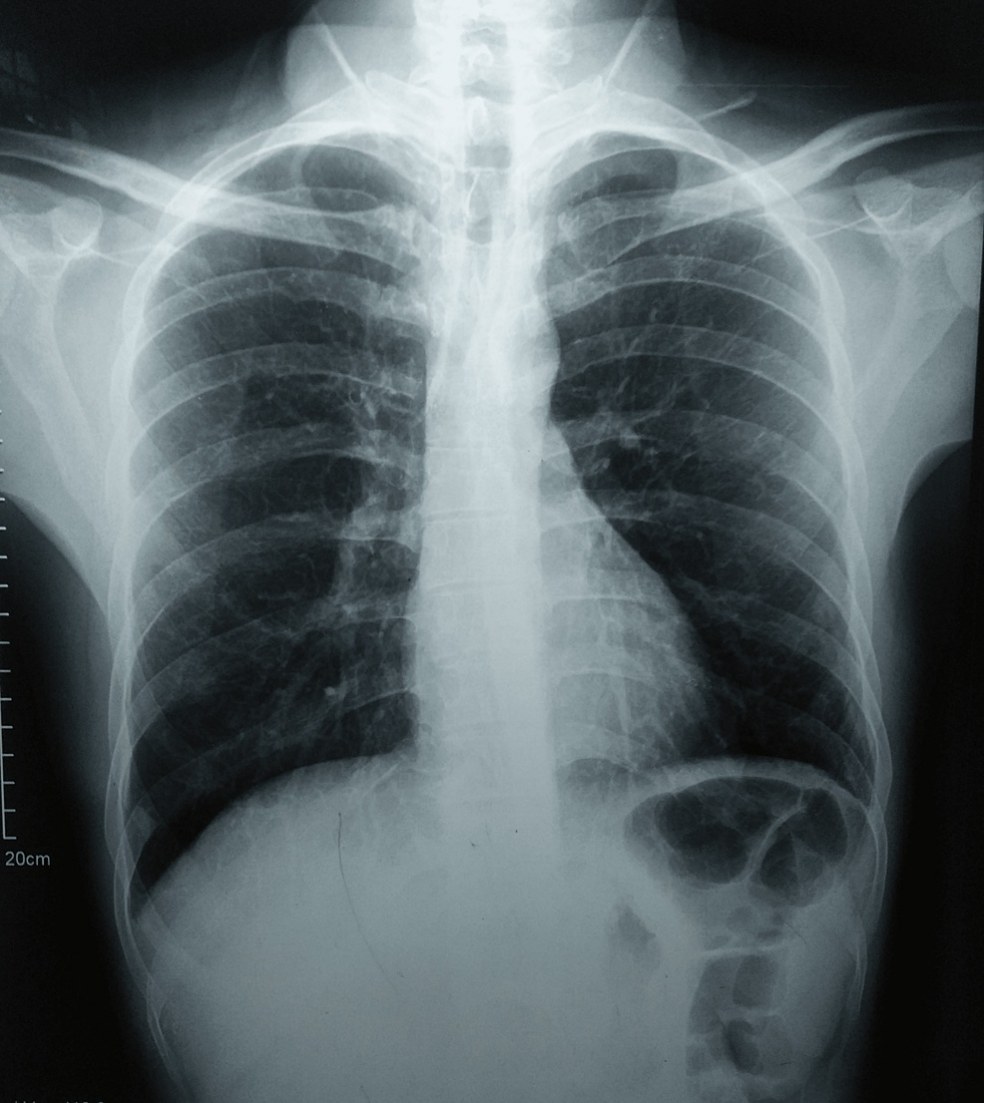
A plain chest radiograph not suggestive of pulmonary involvement
